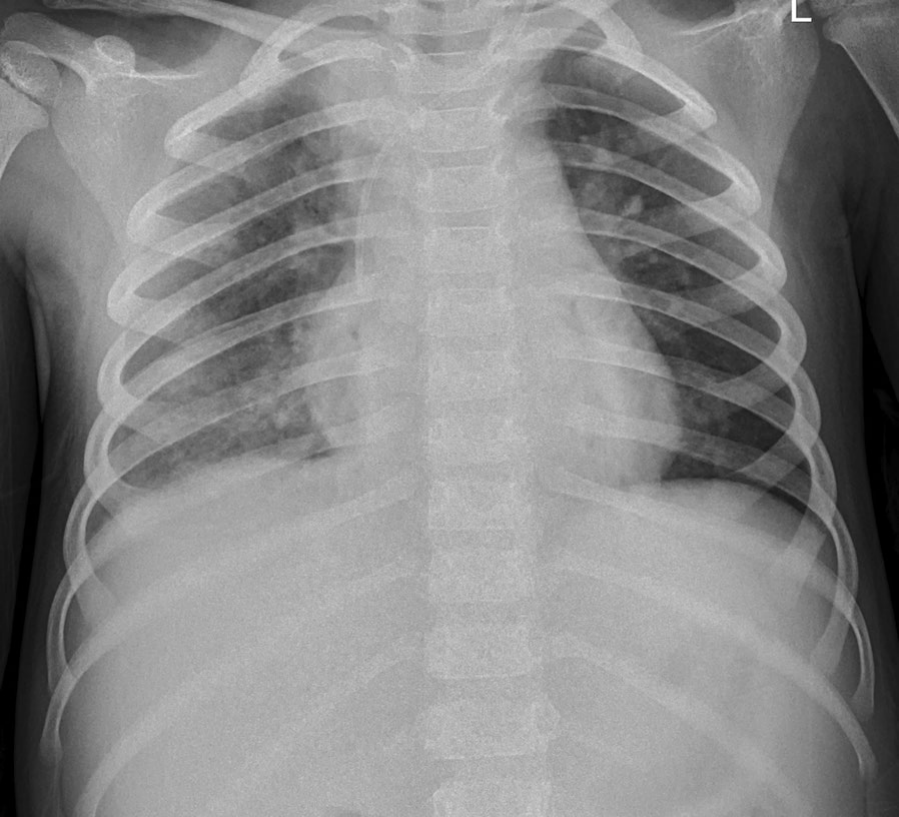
Chest X-ray after stopping ventilator assisted ventilation showed the inflammatory changes of both lungs were significantly improved
